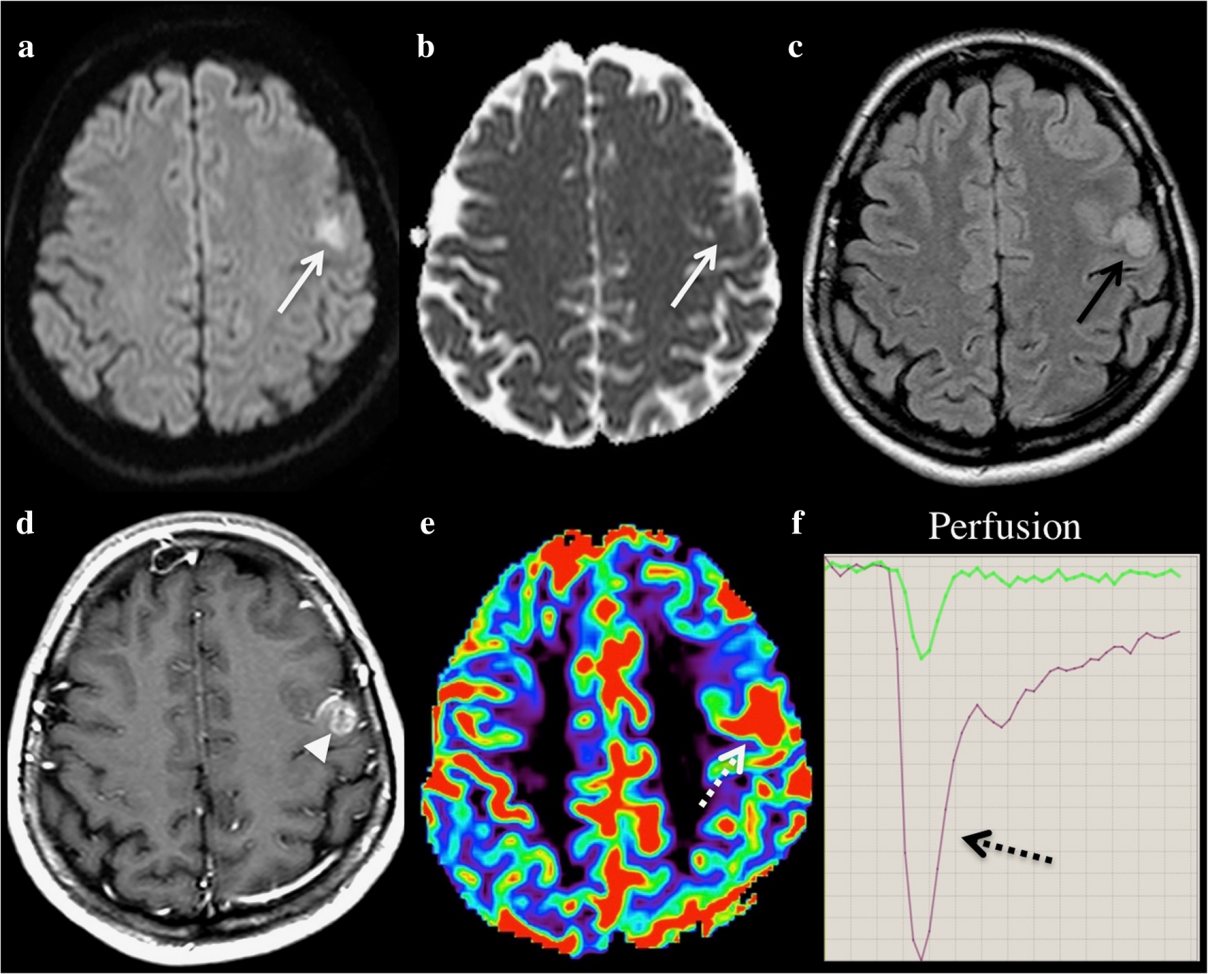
A 54-year-old woman with brain tumour (glioblastoma) presenting with language deficit. DWI shows hyperintensity (a, white arrow) with subtle restriction on ADC (b, white arrow). Intra-axial left frontal mass with hypersignal FLAIR swelling (c, black arrow) and contrast enhancement (d, white arrowhead). Perfusion-weighted imaging (PWI) shows increased cerebral blood volume (CBV) (e, f, dotted arrows)
